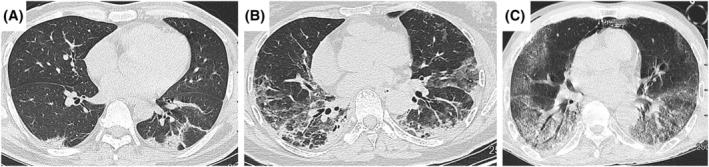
Representative chest HRCT patterns. (A) OP pattern, patchy consolidation and irregular cord opacities distributed predominantly around the basal and peripheral lungs. (B) NSIP pattern, ground glass opacities on both lungs, with traction bronchiectasis. (C) DAD pattern, ground glass opacities diffusely distributed under the pleura of both lungs, with traction bronchiectasis.
